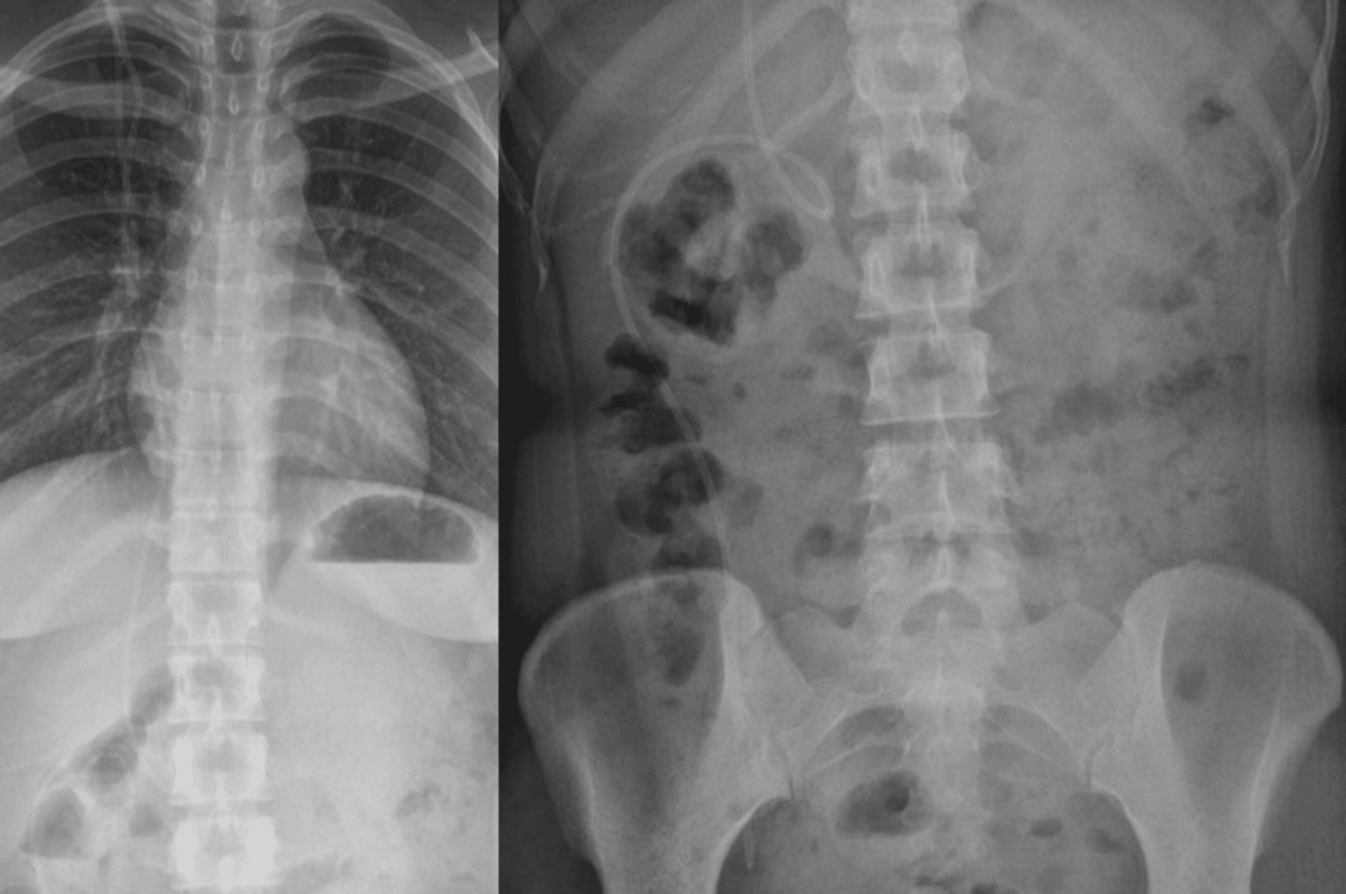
X-ray chest and abdomen to visualize the distal end of the VPS. VPS: ventriculoperitoneal shunt
